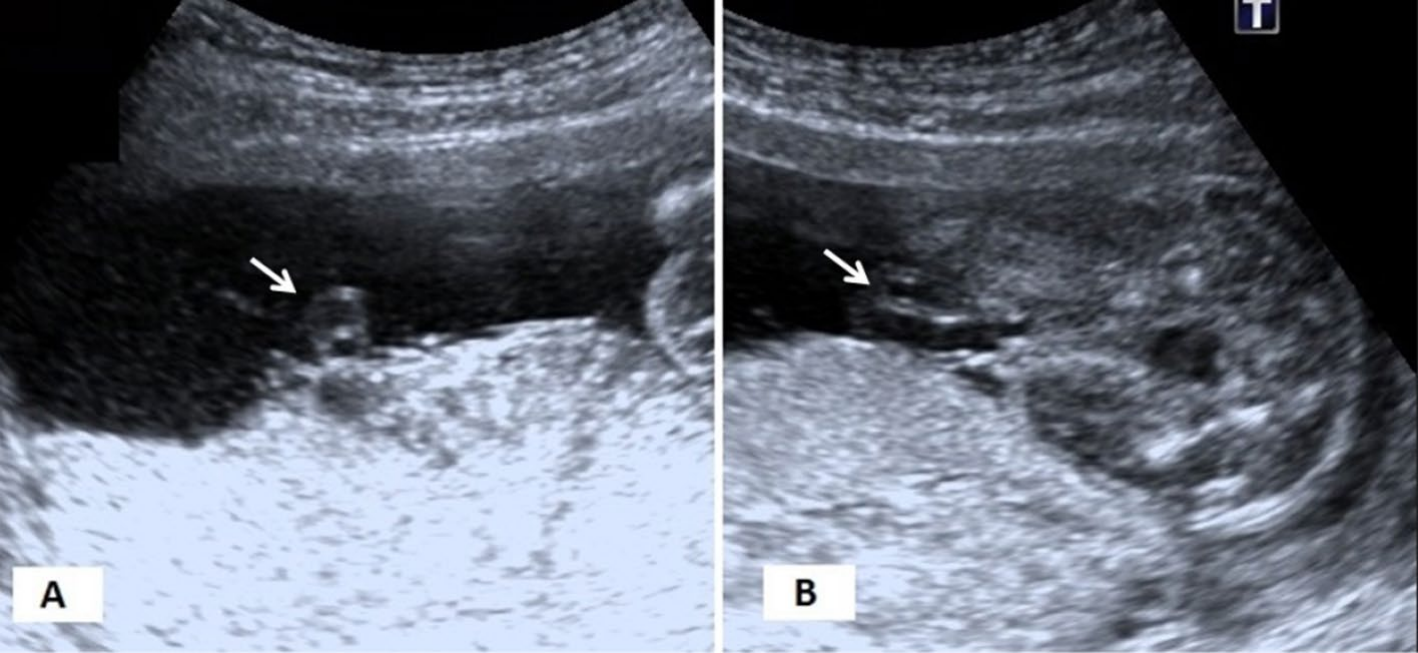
Ultrasound diagnosis of the single umbilical artery is usually performed by evaluation of vessels around the fetal bladder rather than by evaluation of umbilical vessels on (A) axial and (B) longitudinal plane.
